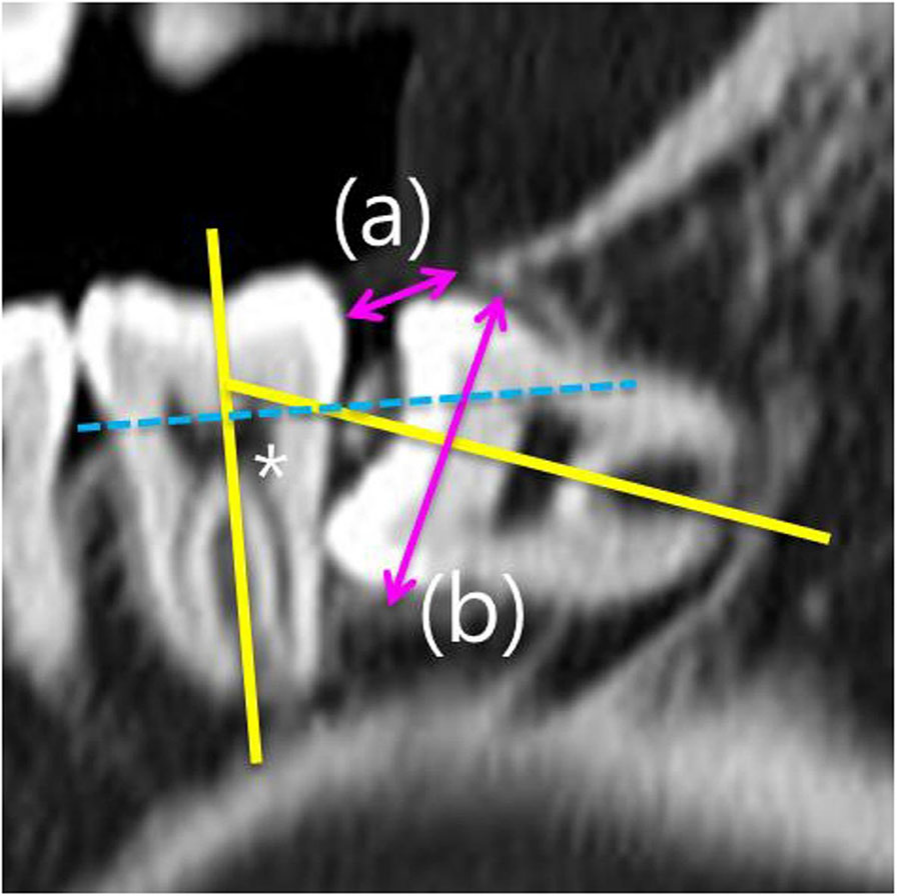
Assessment of the impacted third molar position in computed tomography according to a previous study [8]. The spatial relationship was classified based on the angle measured between the long axis of the impacted third molar and the adjacent second molar (yellow lines and an asterisk mark). Depth was classified based on the line connecting the cementoenamel junction of the adjacent second molar (dotted blue line)
